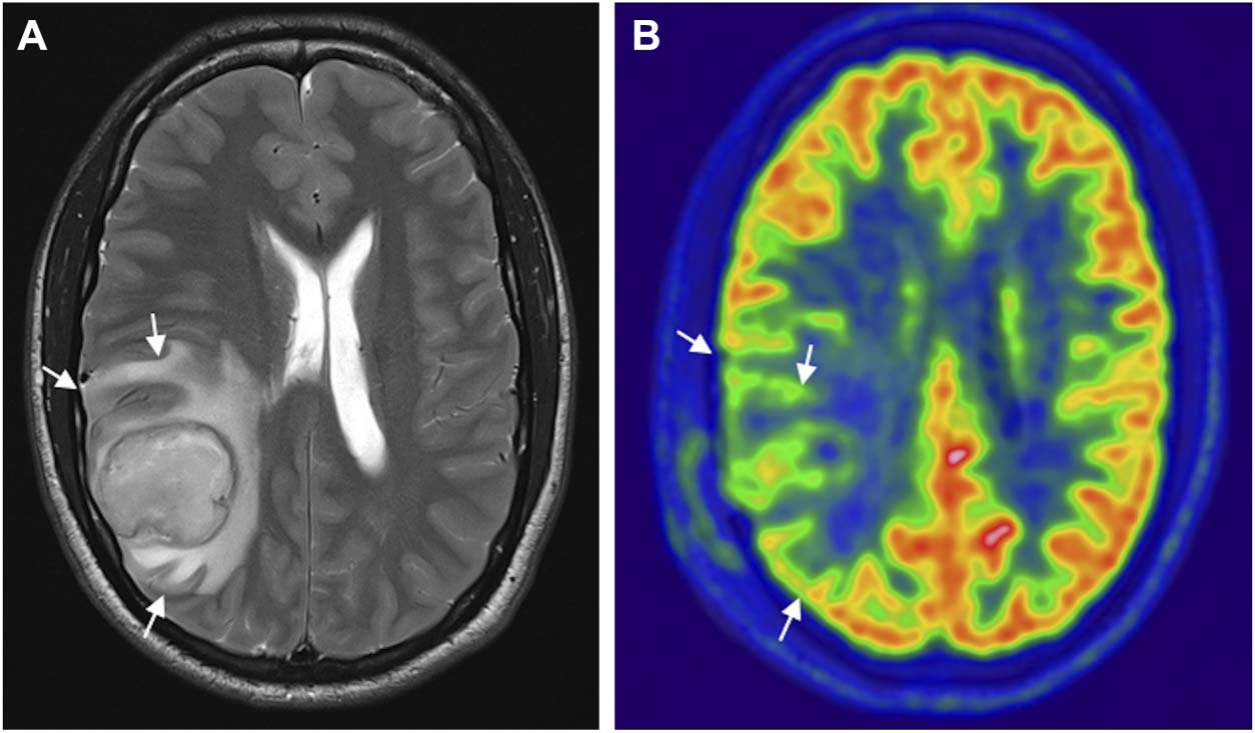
Hypometabolism corresponding to previous peri-abscess vasogenic edema. A, Axial T2-weighted MRI showing an abscess (*) in the right parietal lobe before pus removal. Vasogenic edema surrounds the abscess (arrows). B, Axial [^18^F]-fluoro-2-deoxy-D-glucose positron emission tomography 2 years after pus removal shows neocortical hypometabolism that corresponds to the area previously occupied by the vasogenic edema (arrows).
